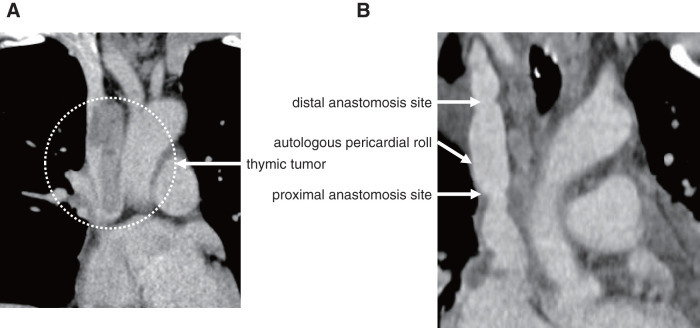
(A) Preoperative computed tomography findings. (B) Postoperative computed tomography findings. (A) The distorted thymic tumor invaded into the SVC and extended into the RAA, as indicated by the white dotted circle. (B) Computed tomography on the 7th postoperative day revealed good patency of the autologous pericardial roll. Both anastomoses of the autologous pericardial roll are indicated by white arrows. SVC: superior vena cava; RAA: right atrial appendage
